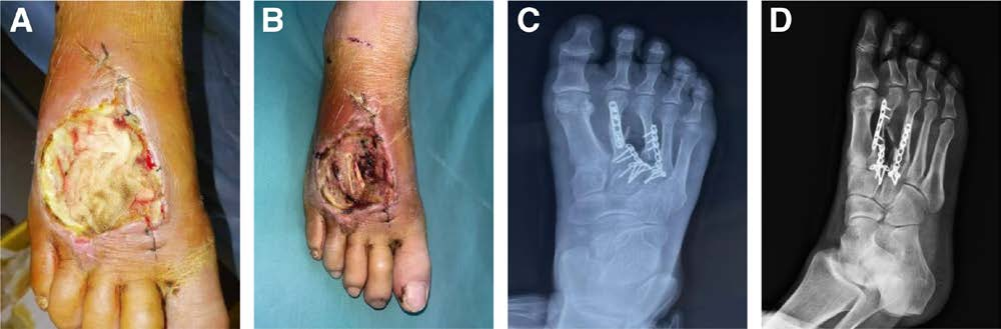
Admission photos. (A) The dorsal foot wound was gauze-filled with purulent exudate. (B) Partial necrosis of the trauma with exposed tendons, bone, and some internal fixation. (C) X-ray: orthostasis after internal fixation. (D) X-ray: oblique position after internal fixation.
